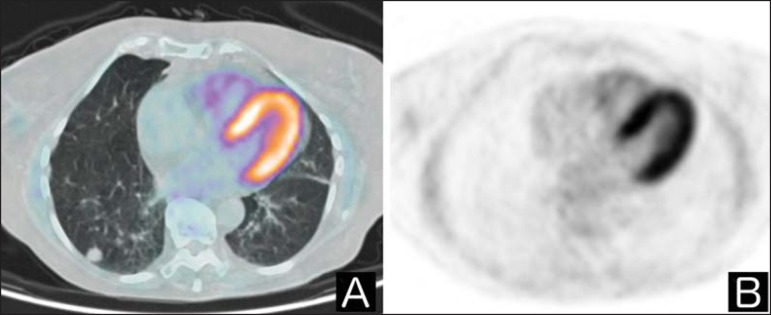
A: Axial fused ^18^F-FDG PET/CT image.
